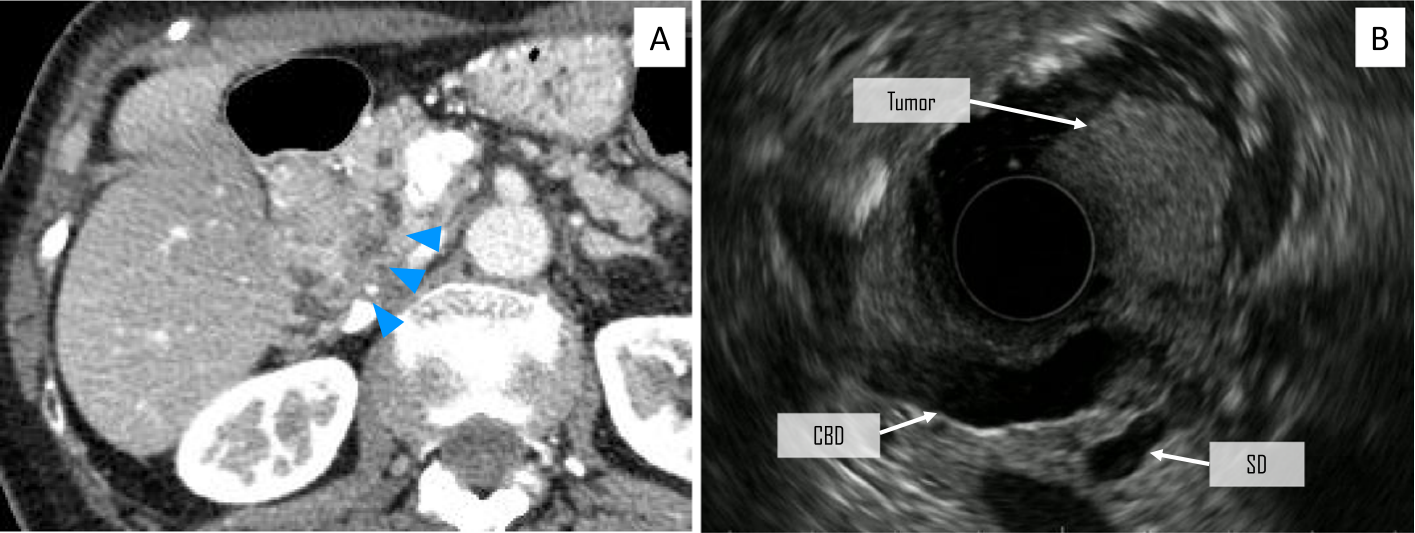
Preoperative imaging studies. Contrast-enhanced abdominal CT imaging demonstrates an enhanced mass in the second part of the duodenum, which is adjacent to the dilated SD (arrowhead) (a). Radial EUS showed that the tumor protruded into the duodenum from the SD (b). CT: computed tomography, EUS: endoscopic ultrasound, SD: Santorini’s duct, CBD: common bile duct
